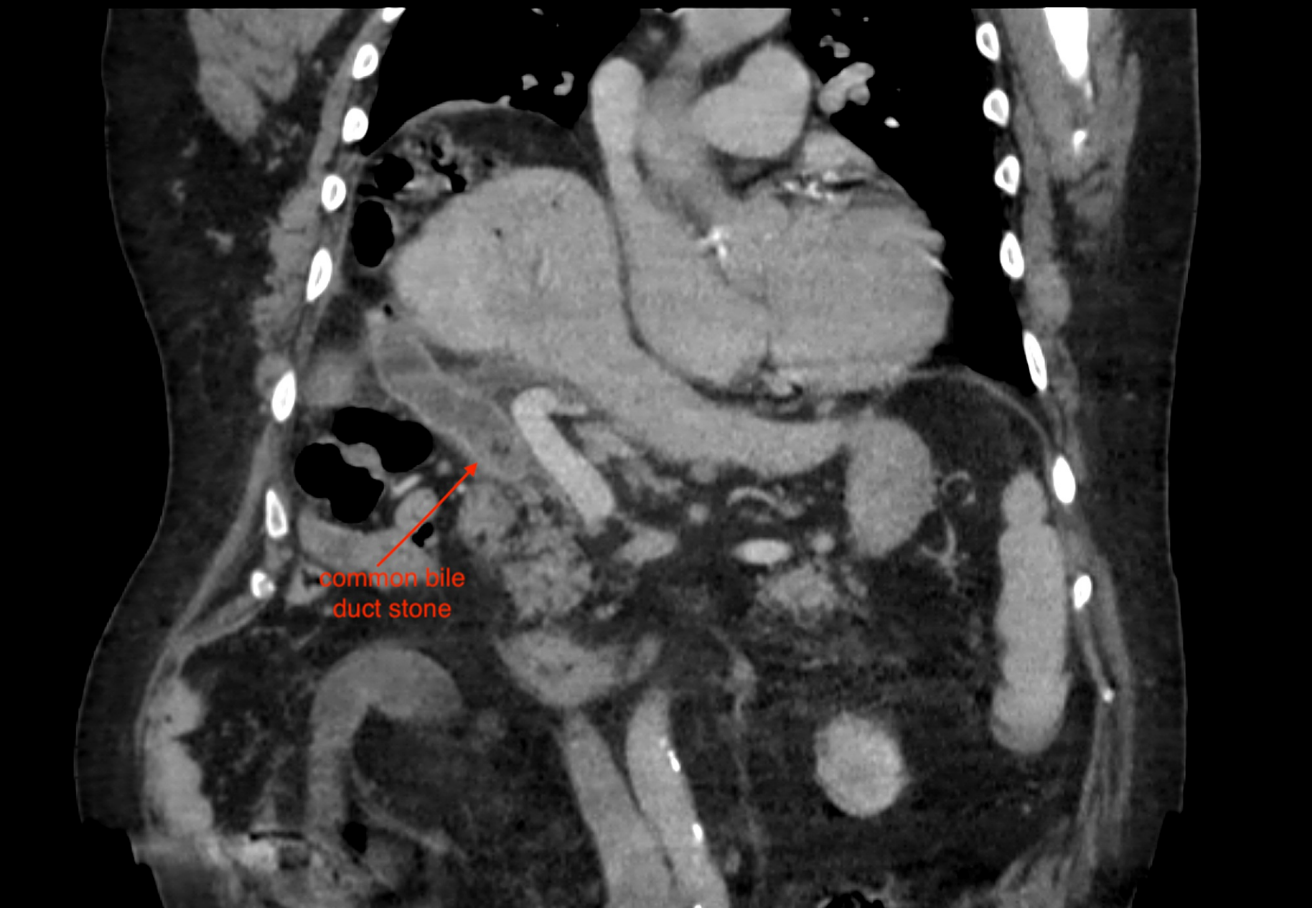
Coronal CT image showing a common bile duct stone
